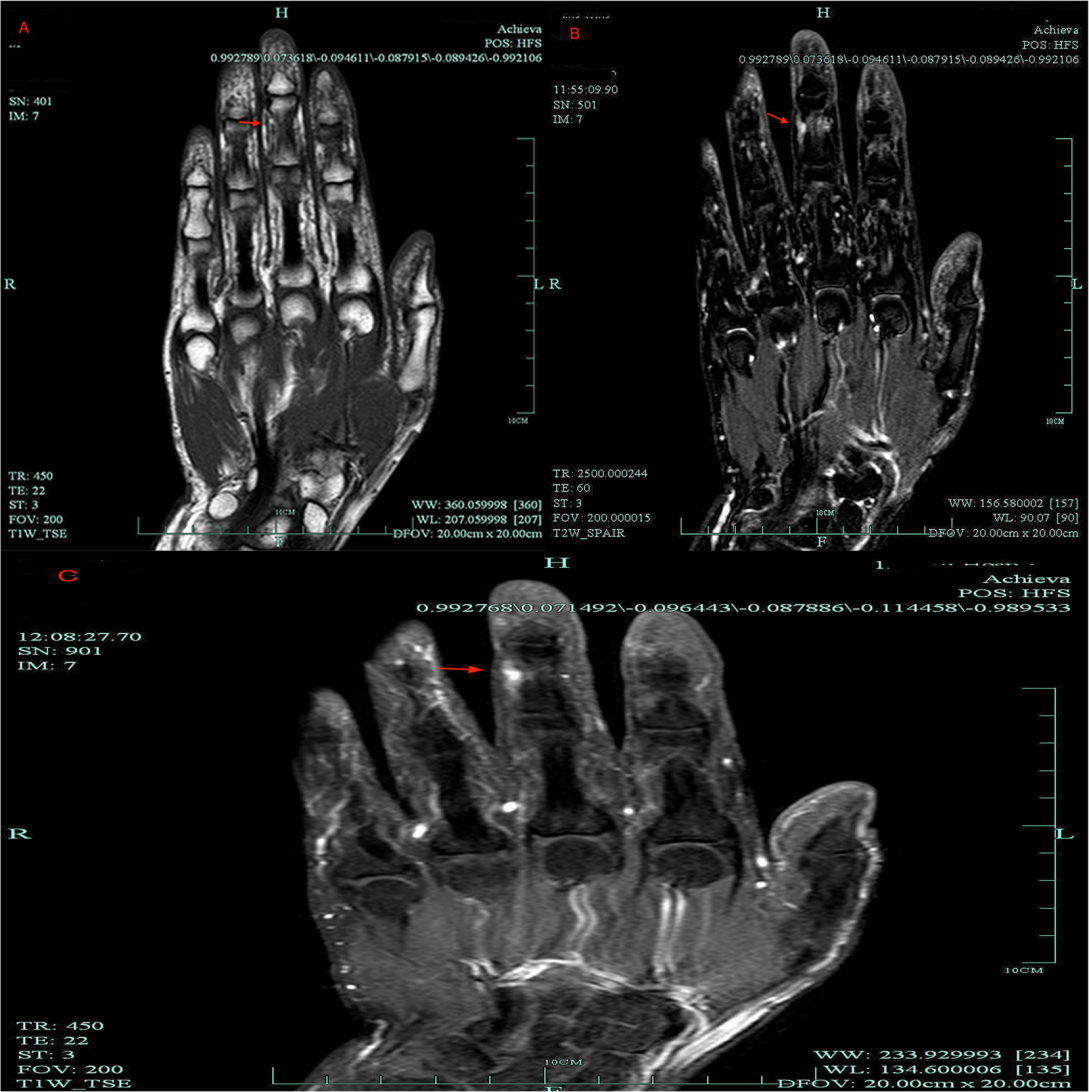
a T1-weighted MRI image showing a hyperintense signal. b T2-weighted MRI image showing a hyperintense signal. c. Contrast-enhanced MRI showing a significantly enhanced signal
